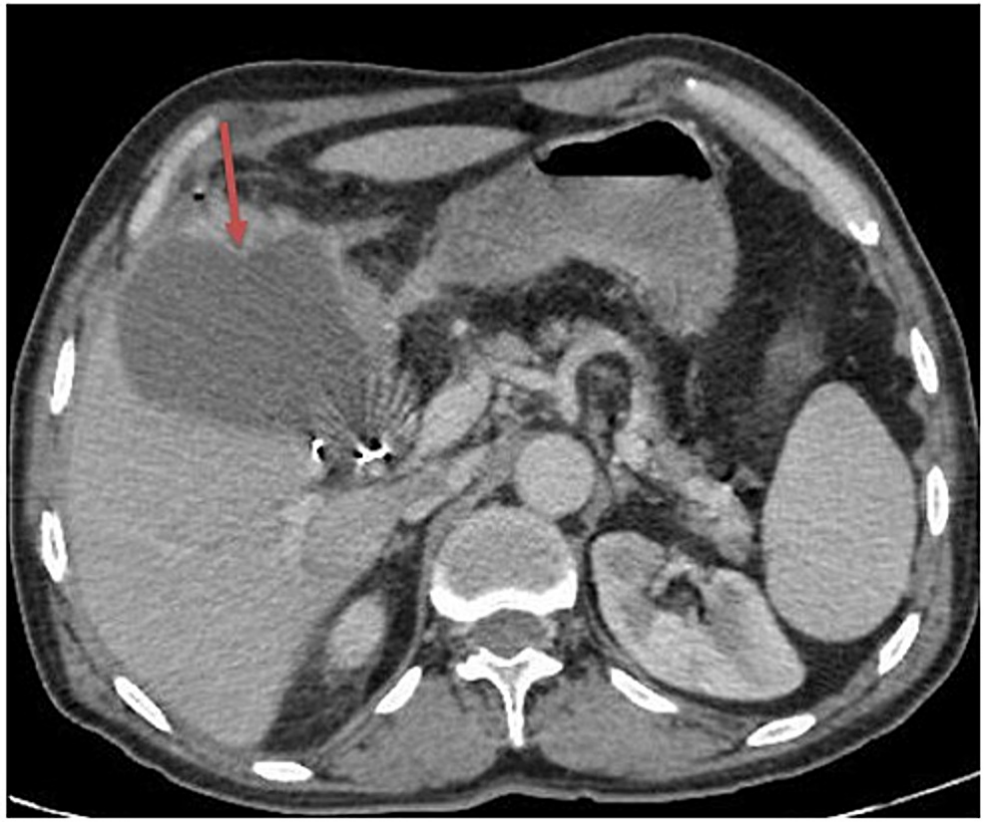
Axial contrast-enhanced CT scan The image shows vesicular bed collection with parietal enhancement (red arrow) CT: computed tomography
